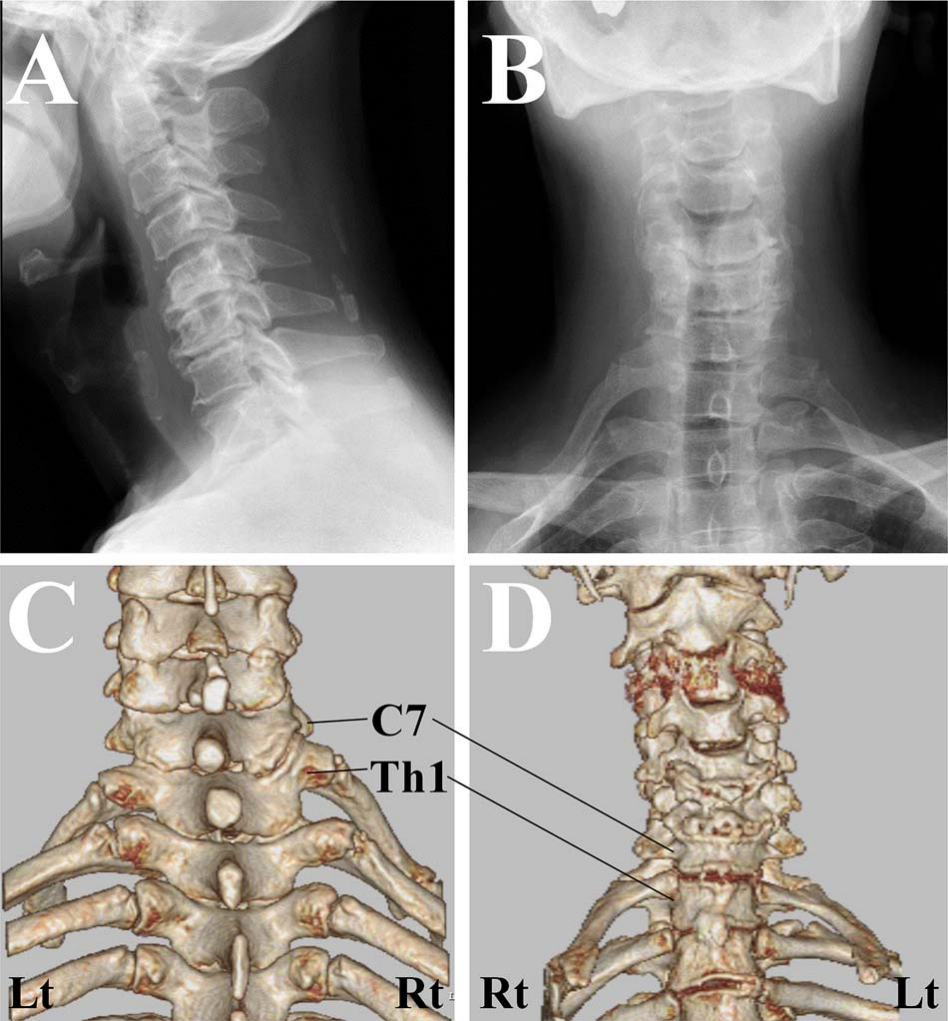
Cervical spine X-ray and computed tomography (CT) images. (A) X-rays image of lateral view showed the straight alignment of the cervical spine. (B) X-rays image of anterior–posterior view showed bilateral sloping shoulders. (C) CT image of posterior–anterior view. The first rib on the right side originated from the higher position of the Th1 vertebra than that on the left side. (D) CT image of anterior–posterior view. CT revealed the existence of the joint between the first rib and the second rib on the right side.
